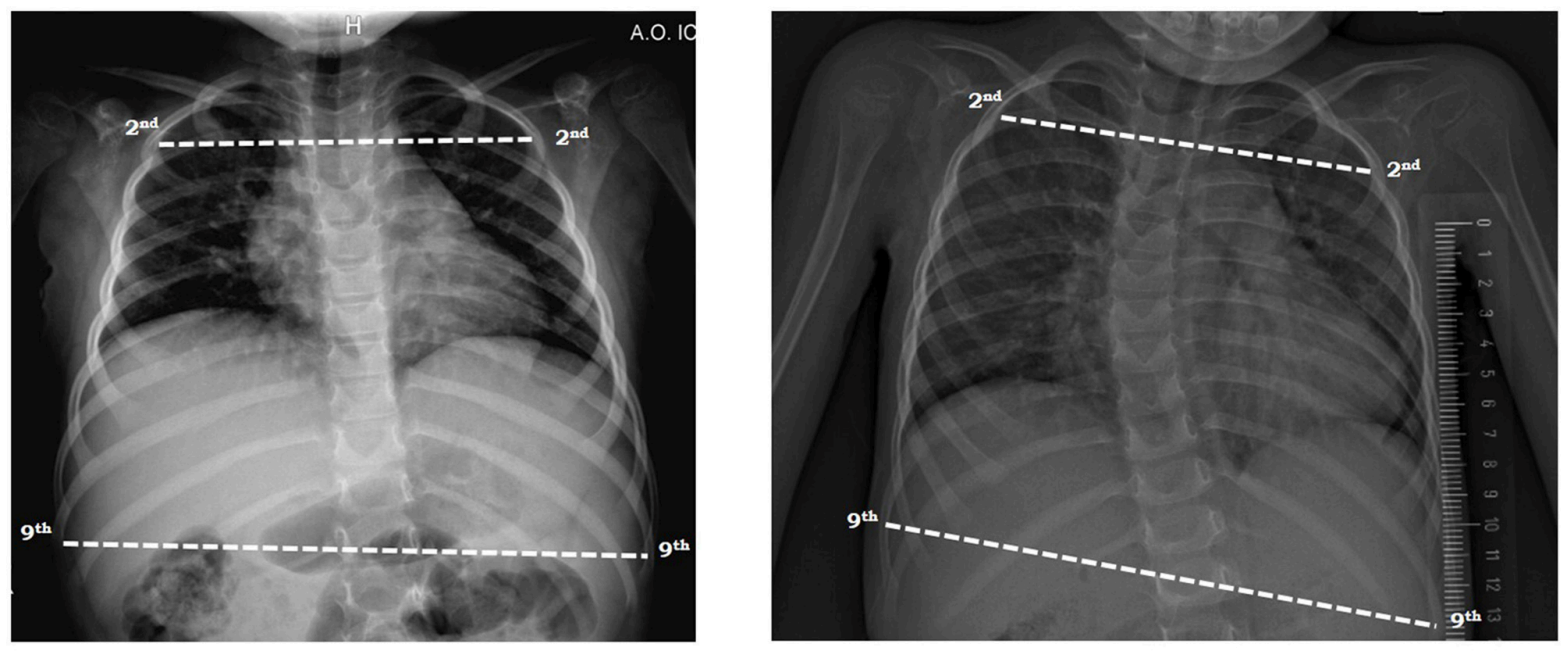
Measurement of the ratio of upper to lower ribcage on antero-posterior view of chest radiograph in a SMA type 2 child with mild (left) and severe (right) scoliosis in supine position. The lines were drawn from the inner margin of the ribs 2 and 9 on one side to the other side.
